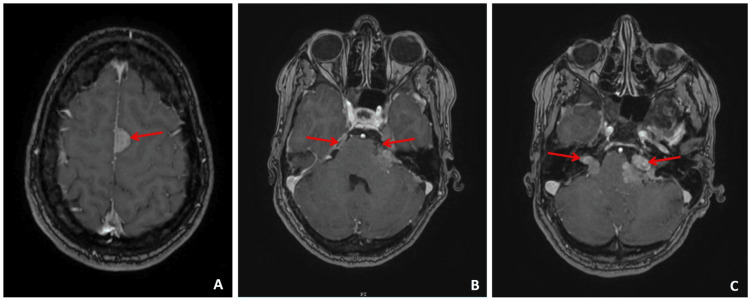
(A) Cerebral MRI axial section T1 sequence injected showing intracranial meningiomas. (B) Cerebral MRI axial section fluid-attenuated inversion recovery (FLAIR) sequence showing bilateral trigeminal neurinoma. (C) Cerebral MRI axial section T1 sequence injected showing bilateral vestibular schwannomas extending into the cerebellopontine cisterns, creating the characteristic "ice cream cone" appearance.
